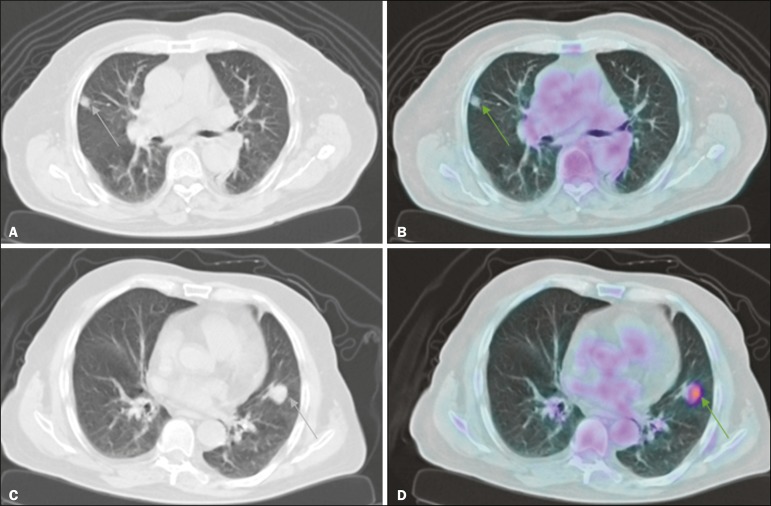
Examples of the use of ^18^F-FDG-PET/CT in SPNs. A: A nodule for which the probability of malignancy was 33.2% on the basis of clinical and CT findings, decreasing to 10% when the absence of ^18^F-FDG uptake was taken into account (a follow-up CT scan at two years out showed that the nodule had disappeared). B: A nodule for which the probability of malignancy was 6.2% on the basis of clinical and CT findings, increasing to 75.8% when the moderate ^18^F-FDG uptake was taken into account (a subsequent biopsy revealed an adenocarcinoma).
